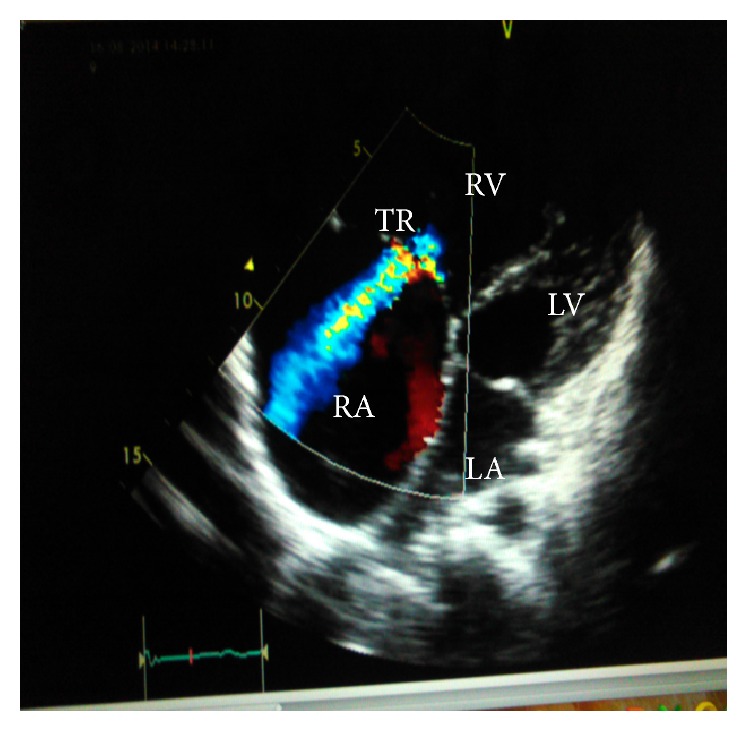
A 4-chamber view with color Doppler showing the tricuspid regurgitation (TR), enlarged right atrium (RA), enlarged right ventricle (RV), compressed left atrium (LA), and compressed left ventricle (LV) before adenotonsillectomy.
